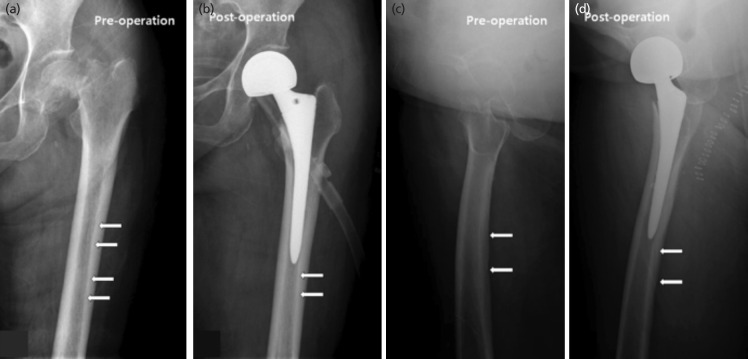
Radiolucent line at the same position as on pre-operative and post-operative radiographs. (a) Nutrient artery canal (arrow) on pre-operative anteroposterior view. (b) Radiolucent line (arrow) on post-operative anteroposterior view. (c) Nutrient artery canal (arrow) on pre-operative translateral view. (d) Radiolucent line (arrow) on post-operative translateral view.
